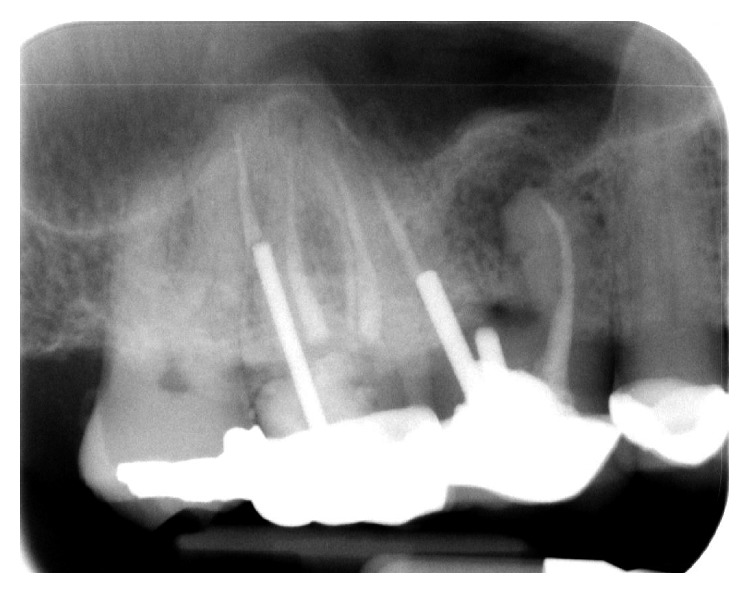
Radiograph of tooth #3 showed periapical radiolucency on the mesial buccal root.
